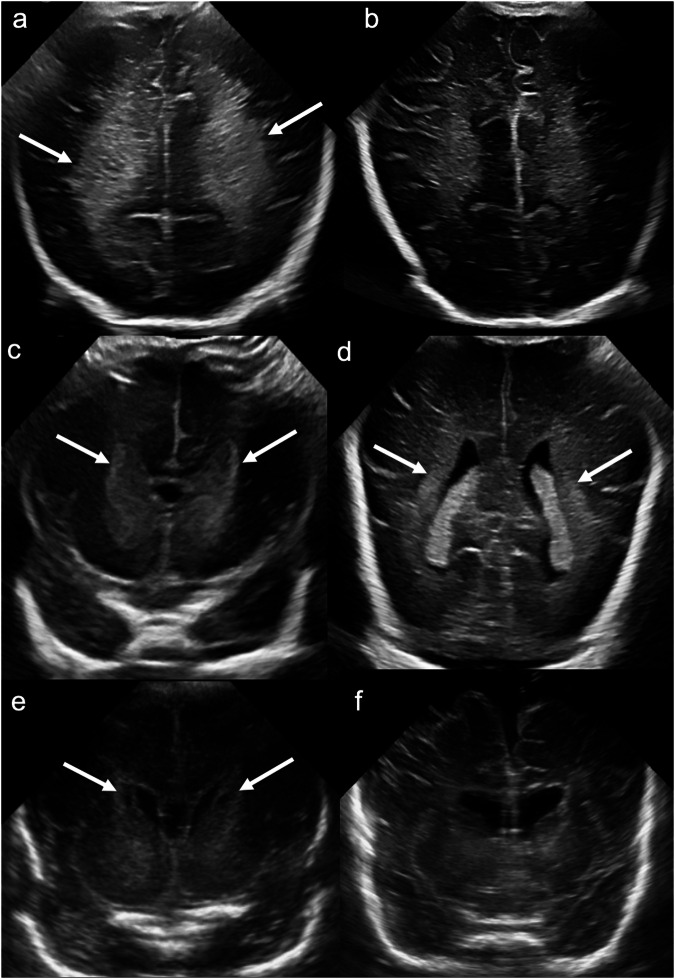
Periventricular flare. a Coronal image acquired with a medium-frequency (3–10 mHz) curved probe at the level of the parietal and occipital lobes on day 1 of life in a preterm infant demonstrates bilateral, symmetrical, homogenous increased echogenicity of the posterior white matter (solid arrows). b Coronal image at the same level on day 28 ultrasound demonstrates normalisation of parieto-occipital white matter echogenicity. Term-equivalent MRI (not shown) demonstrated normal periventricular white matter signal, without cystic change. Normal white matter tracts. c, d Coronal images acquired with a medium-frequency (3–10 mHz) curved probe at the level of: (c) the lateral ventricular frontal horns and (d) the lateral ventricular trigones demonstrate bilateral, symmetrical, increased echogenicity along the anterior limbs of internal capsules (c) and optic radiations (d) respectively (solid arrows), consistent with normal white matter tracts. Connatal cysts. e Coronal image acquired with a medium-frequency (3–10 mHz) curved probe at the level of the frontal horns demonstrates bilateral periventricular cysts situated anterior to the foramina of Monro and below the superior margin of the frontal horns (solid arrows). f Coronal image at the same level on an ultrasound performed at 4 months corrected age (for increasing head circumference) demonstrates resolution of the periventricular cysts
